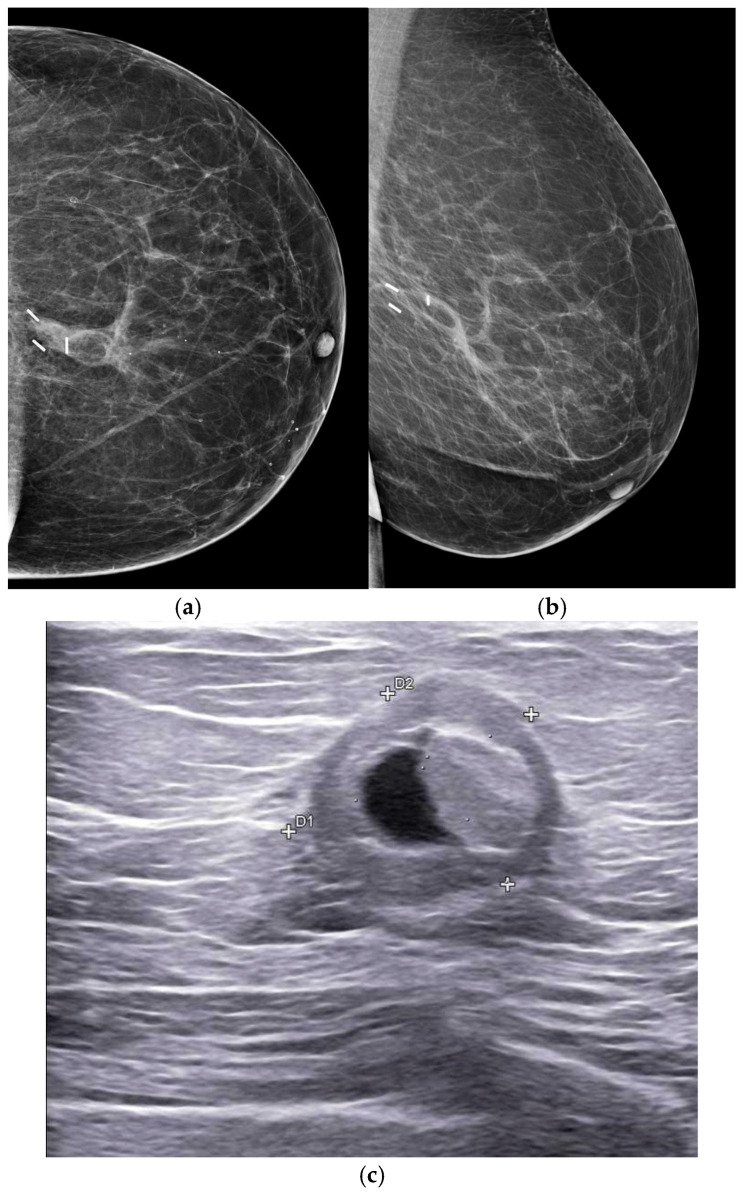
A 58-year-old woman undergoing follow-up at the completion of treatment, one year after surgery and radiotherapy. Features consistent with fat necrosis are present, characterized mammographically by a radiolucent area with thin, peripheral dystrophic calcifications (a,b). Fat necrosis typically results from adipose tissue injury and evolves through inflammatory and fibrotic stages. On ultrasound, the finding corresponds to a well-defined liponecrotic cyst containing internal echoes or mixed echogenic material, reflecting liquefaction of necrotic fat (c).
